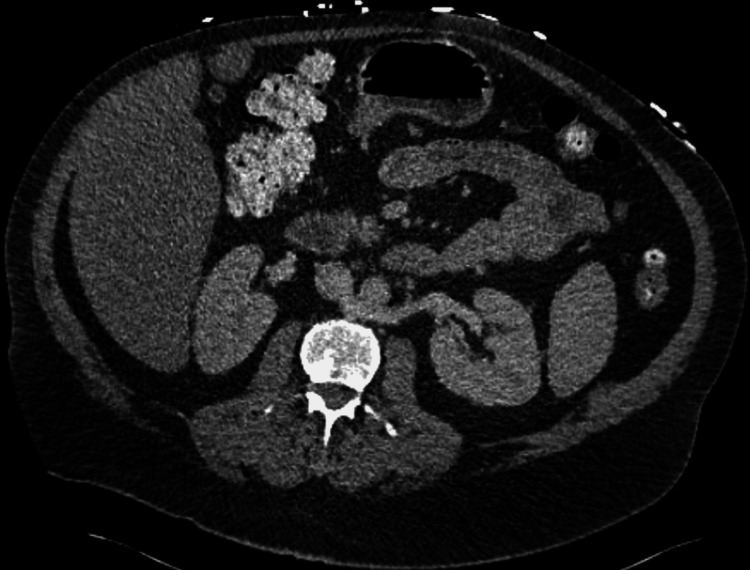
CT abdomen pelvis at admission with no acute abnormality
